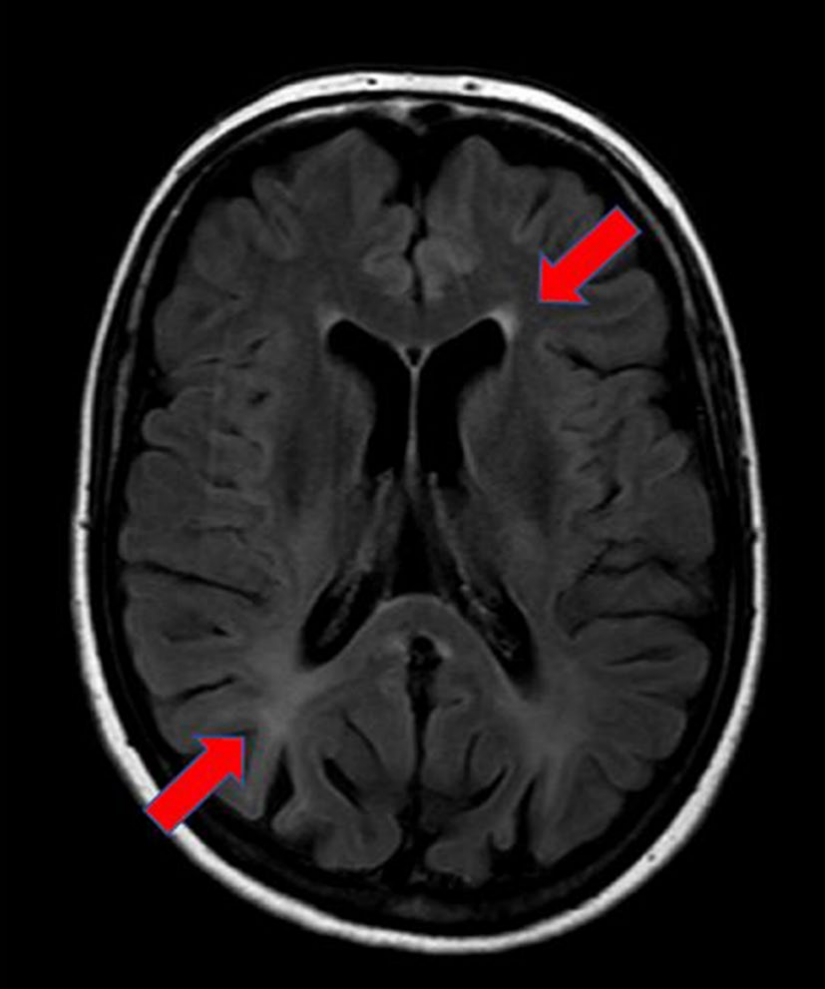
MRI (FLAIR) of a 50-year-old patient with PKU who was early diagnosed and treated but discontinued diet at the age of 14. Arrows point to periventricular white matter hyperintensities, a sign of metabolic (e.g. PKU-related) encephalopathy
